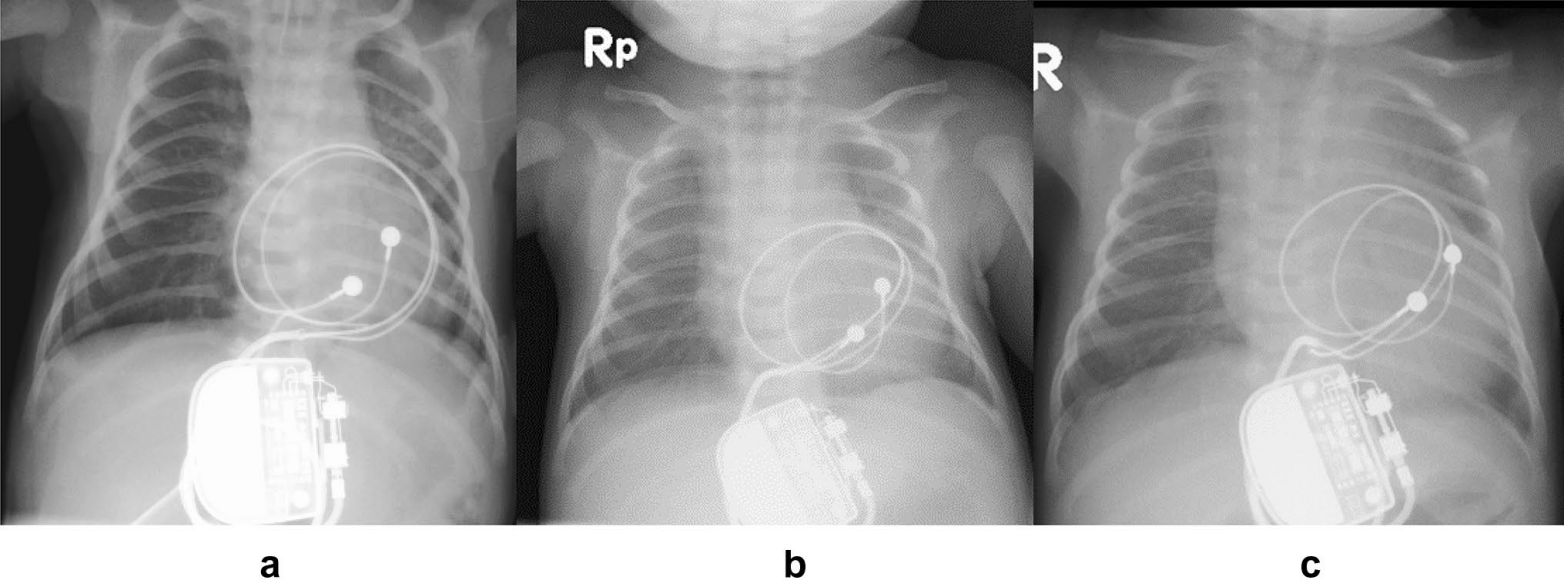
Serial of chest radiographs a just after the first implantation of the generator at 6 days of age b at postoperative 14th day which might indicate that the leads have already slid beside the main pulmonary artery, and c at postoperative 16th day
